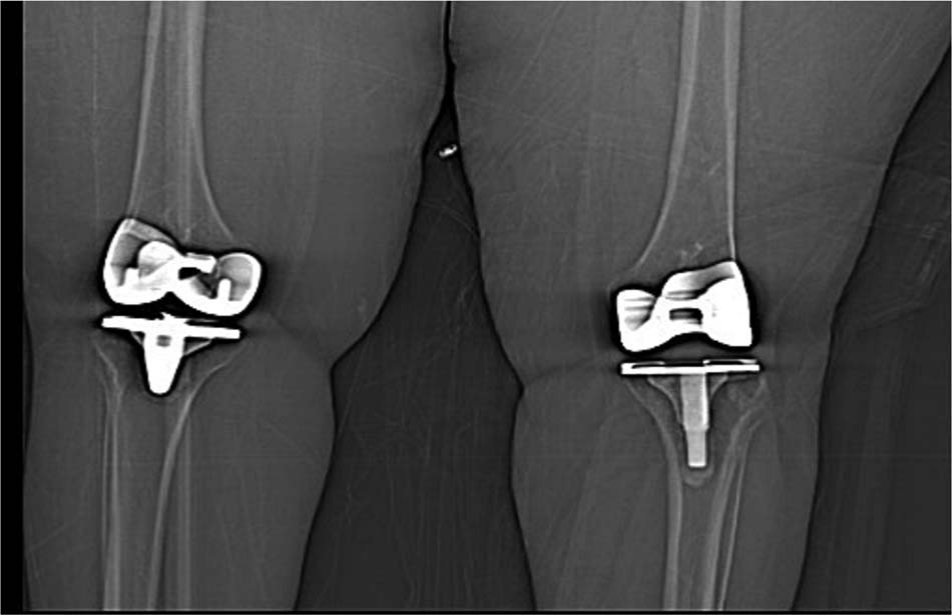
Computed tomography (CT scan) of bilateral knees showing no abnormality.
